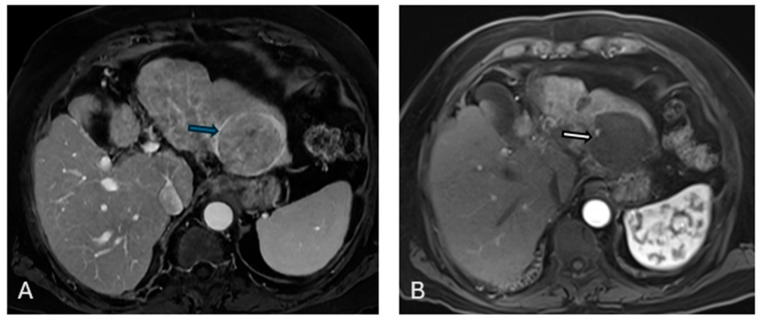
Axial contrast-enhanced magnetic resonance imaging (MRI) demonstrating treatment response after radiation segmentectomy. (A) Pre-treatment image shows a large arterially enhancing mass (arrow) consistent with hepatocellular carcinoma (HCC). (B) Post-treatment follow-up demonstrates necrosis and lack of enhancement within the treated segment (arrow), consistent with successful radiation segmentectomy.
